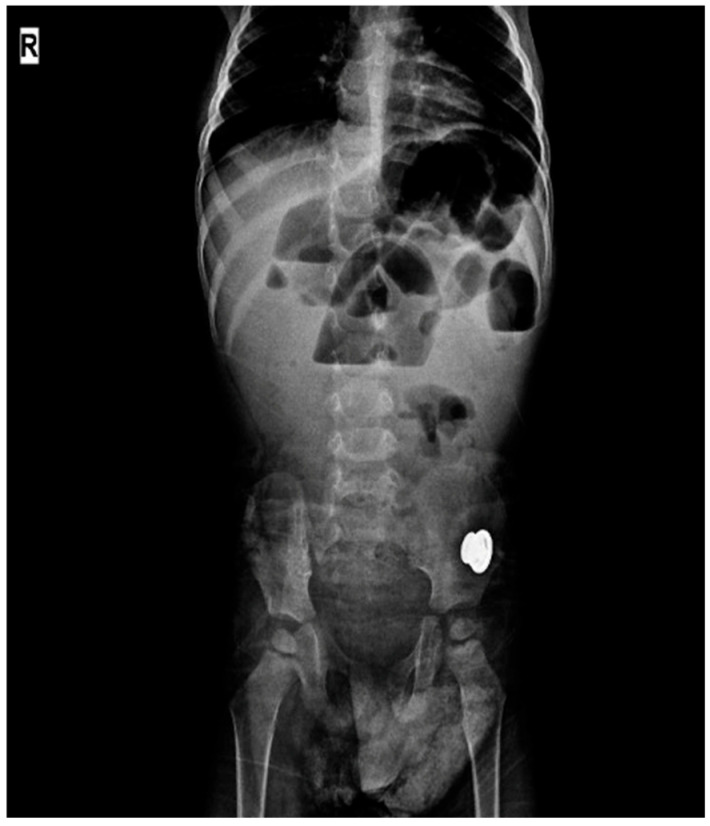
Abdominal X-ray image of a patient presenting with Hirschsprung-associated enterocolitis (HAEC). Disseminated air-fluid levels are present.
